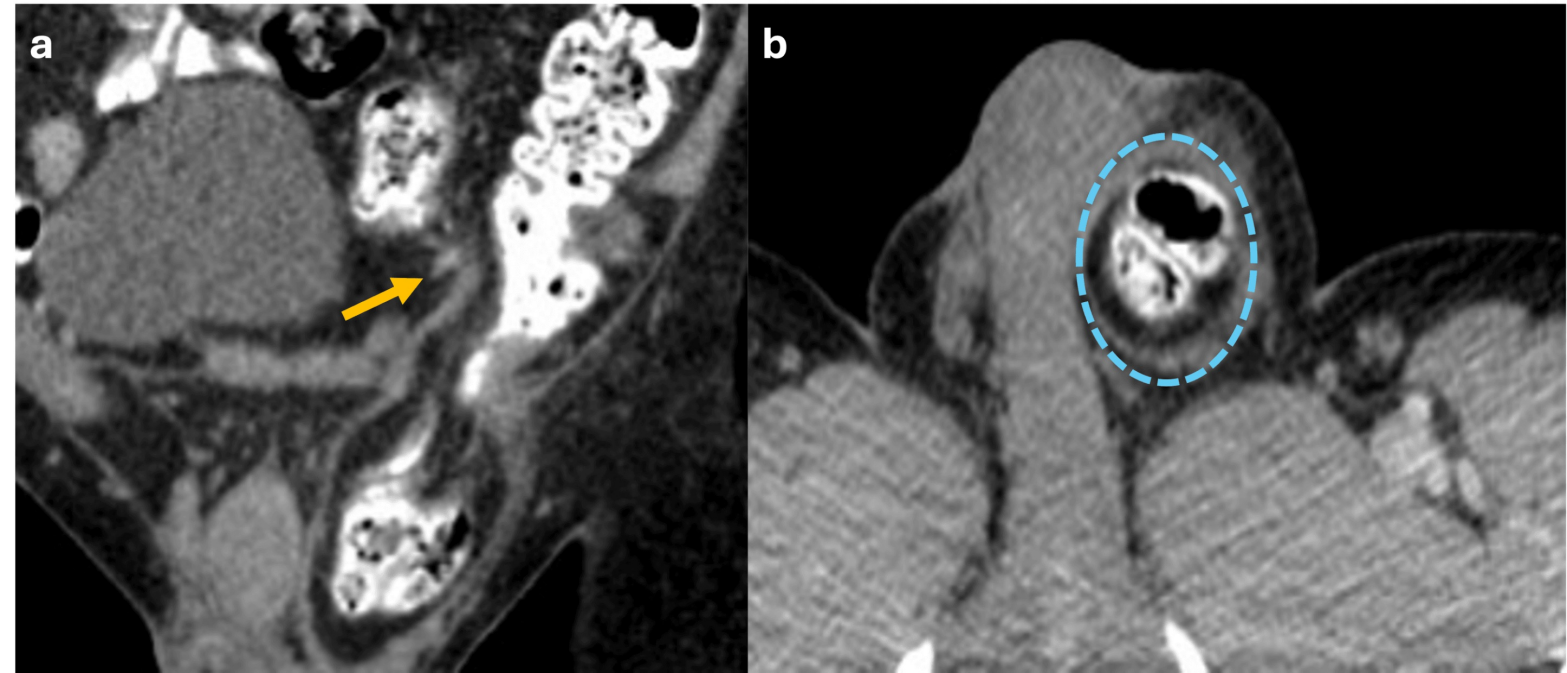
Coronal and axial CT scan of the abdomen with oral contrast showing an indirect inguinal hernia (a) Coronal CT scan of the abdomen with oral contrast revealing an indirect inguinal hernia positioned laterally to the inferior epigastric vessels (yellow arrow). (b) Axial CT scan showing that the hernial sac within the inguinal canal contains part of the sigmoid colon (blue dotted circle). The figure was created by the authors.
